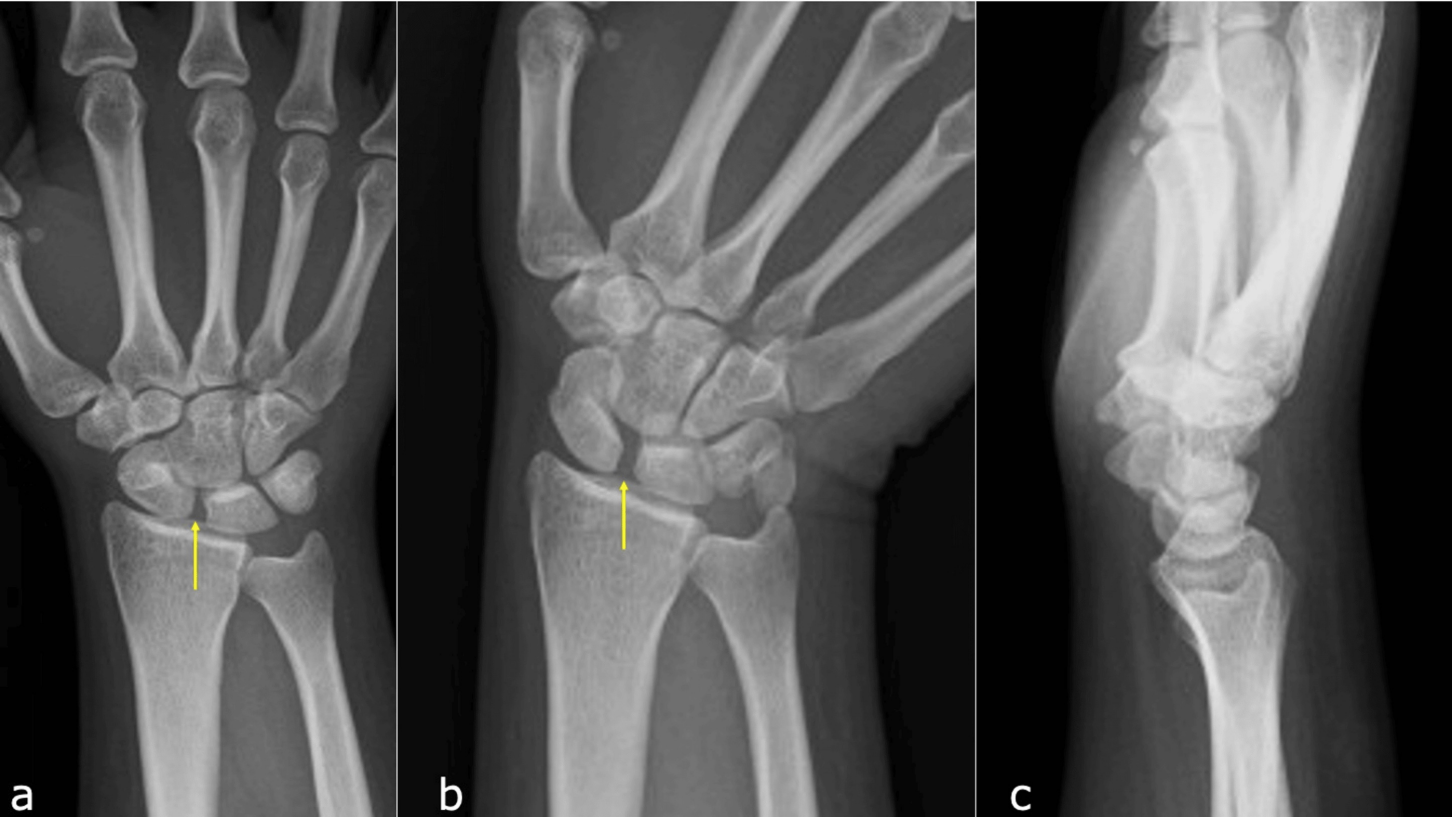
Initial X-ray at hospital admission. a: Anteroposterior (AP) view. b: AP view with ulnar deviation showing widening of the scaphoid-lunate interval (arrow). c: Lateral view, showing a radio-scaphoid angle of 40°.
